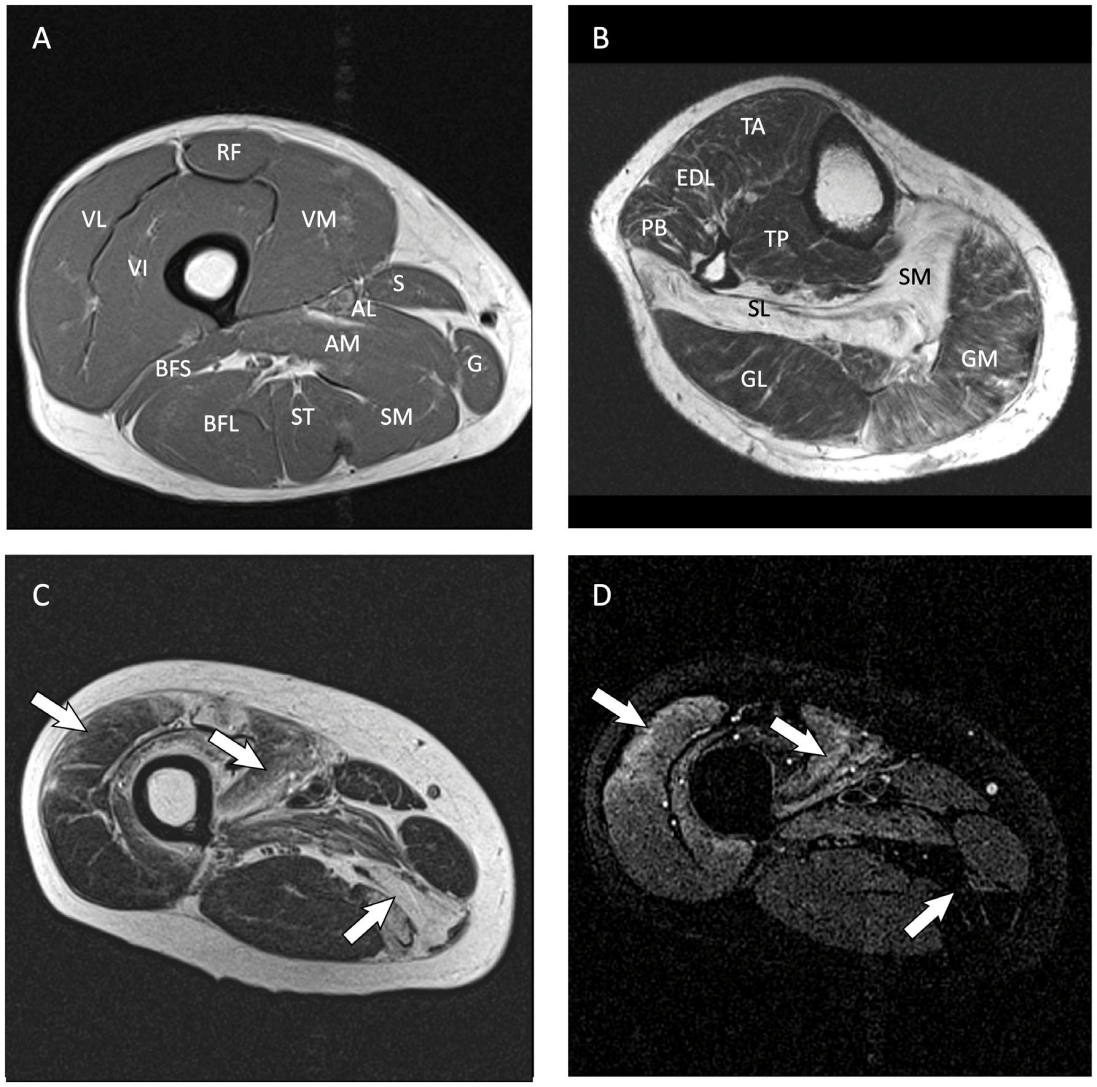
Typical transversal T1 weigthed and TIRM MR images of FSHD patients. (A) Transverse T1 weigthed image of the thigh of a male FSHD patient (age 38), showing fatty infiltration (hyperintense signal) in the semimembranosus and semitendinosus muscles. (B) Transverse T1 weighted image of the leg of a male FSHD patient (age 66 year). Fatty infiltration of the soleus muscles is clearly visible. (C) Transverse T1 weighted image of the thigh of a 39-year-old male FSHD patient. (D) Corresponding TIRM image. The semi-membranosus is clearly fat infiltrated (grey striped arrow), this results in a nulled signal on the corresponding TIRM image. In contrast, the vastus lateralis and vastus intermedius show hyperintense signal in the TIRM images (white arrows) reflecting edema or inflammation. The different muscles in the thigh (Fig. 1.A) and calf (Fig. 1.B) are indicated by the following abbreviations: rectus femoris (RF), vastus lateralis (VL), vastus intermedius (VI), vastus medialis (VM), sartorius (S), adductor longus (AL), adductor magnus (AM), gracillis (G), semi membranosus (SM), semi tendinosus (ST), biceps femoris long head (BFL) and biceps femoris short head (BFS), tibialis anterior (TA), extensor digitorum, longus (EDL), peroneus brevis (PB), tibialis posterior (TP), soleus medialis (SOM), soleus lateralis (SL), gastrocnemius medialis (GM) and gastrocnemius lateralis (GL).
